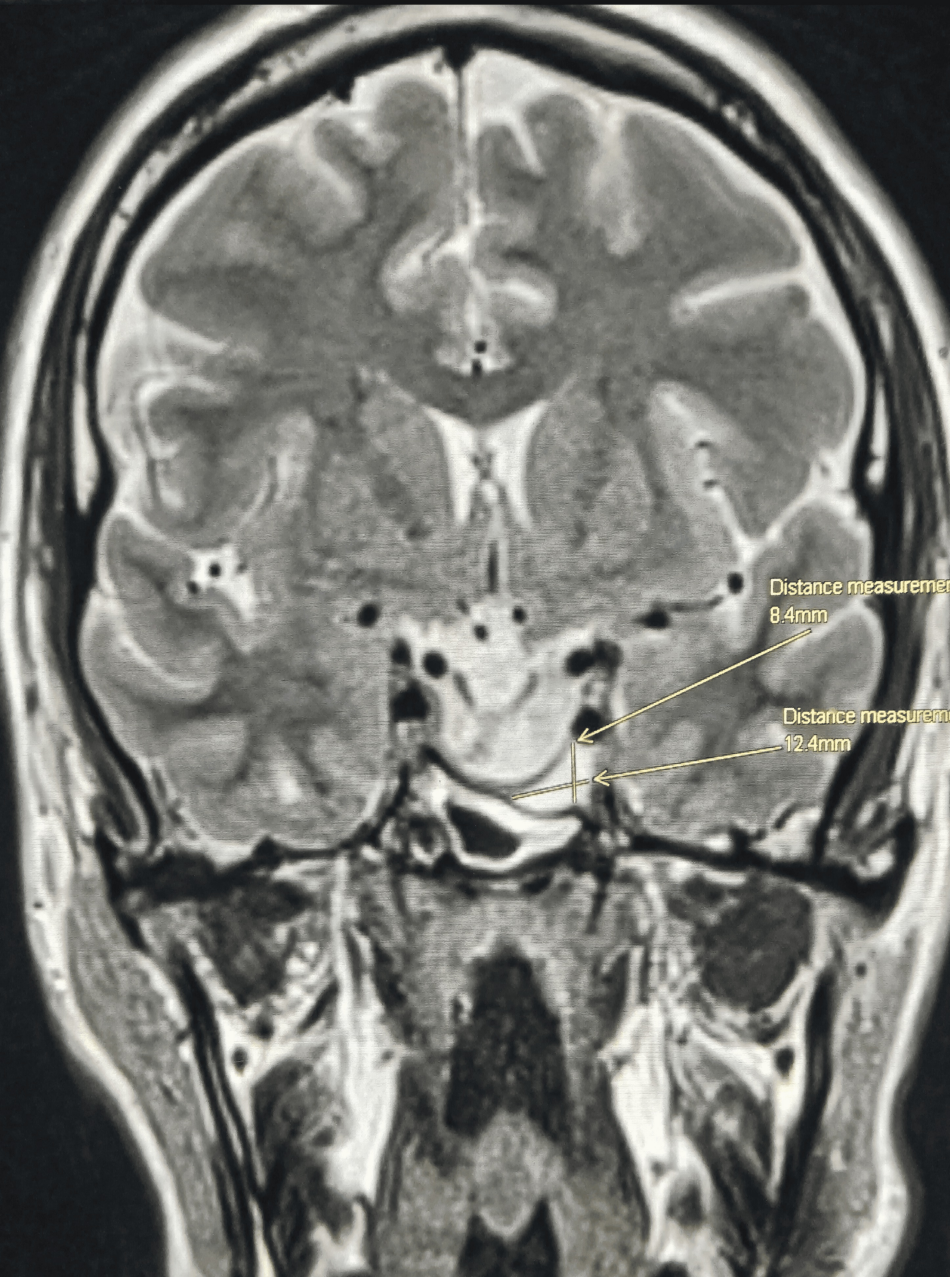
Pituitary MRI - coronal view Twelve months after treatment, with the dimensions of lesion.
